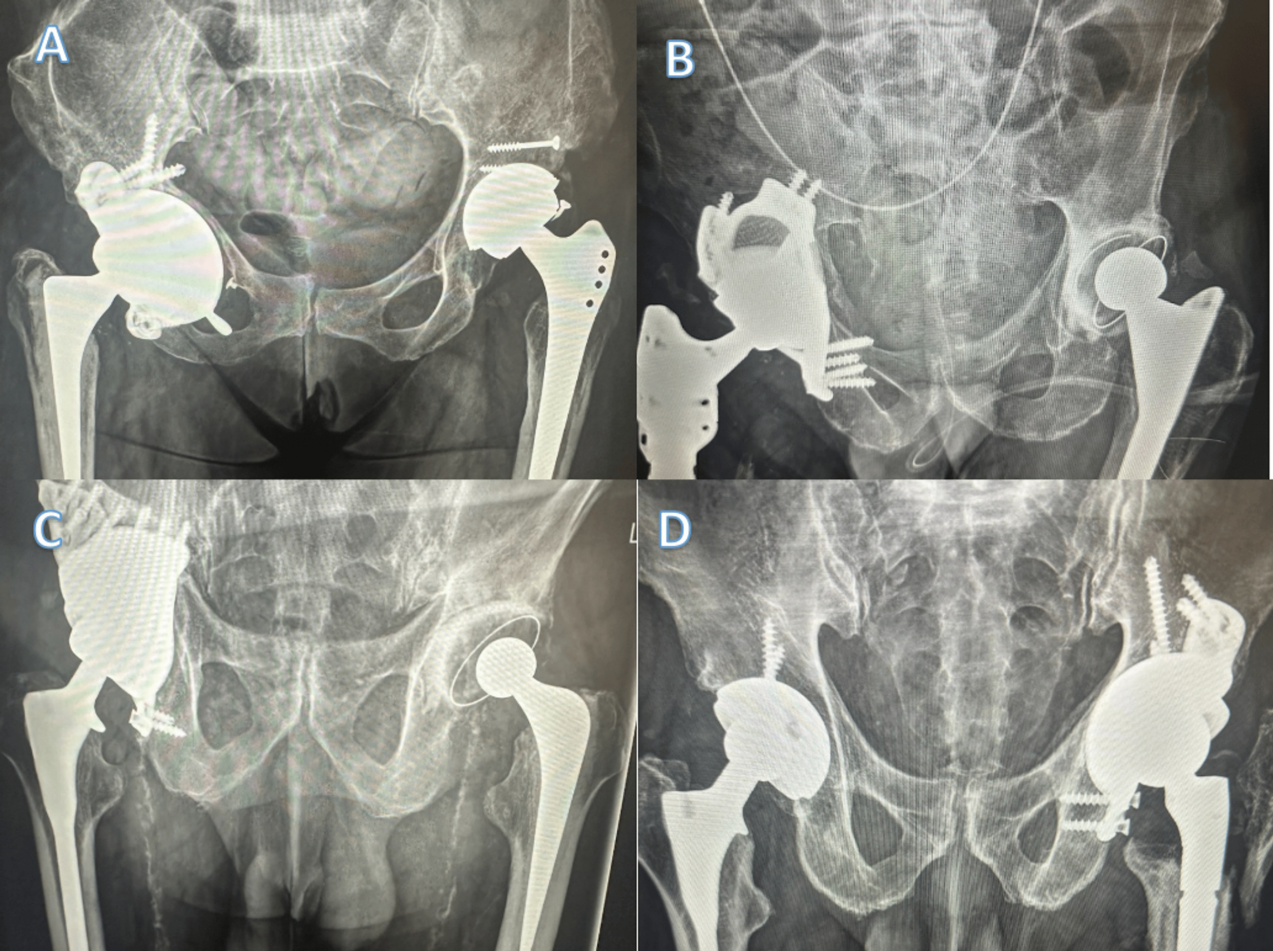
Examples of Postoperative Custom Hip Replacements A: A 56-year-old female with a background of developmental hip dysplasia had a revision of a previous right THR due to anterolateralisation of the component and acetabular bone loss. Nine-month radiographs show osteointegration. B: An 84-year-old male with a background of rheumatoid arthritis had recurrent hip infection and had a right CAI on the second-stage operation. Six-month radiographs show osteointegration. C: A 79-year-old male who had a previous road traffic accident, then had two previous right THRs due to degenerative joint disease, had a right CAI due to pain and acetabular component loosening. Six-month radiographs show osteointegration. D: An 86-year-old male had a fall onto the left THR sustaining a periprosthetic acetabular fracture with PD, thus needing a left CAI for reconstruction. Seven-month radiographs show osteointegration. THR: total hip arthroplasty; CAI: custom acetabular implant; PD: pelvic discontinuity
